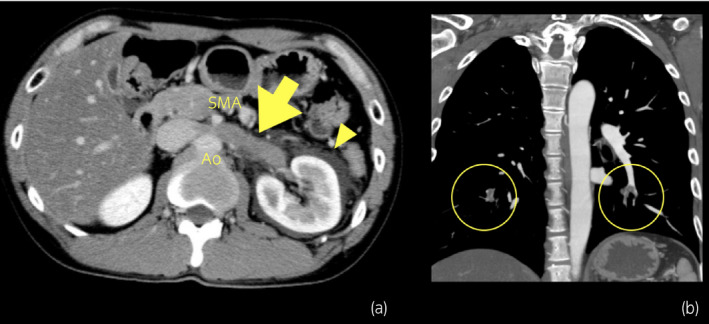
CTA revealed compression of the left renal vein between the aorta and SMA with a left RVT (yellow arrow). Increase in CT‐value around the left kidney indicates congestion by the thrombus (arrow head) (a). CTA revealed bilateral PTE (b).
